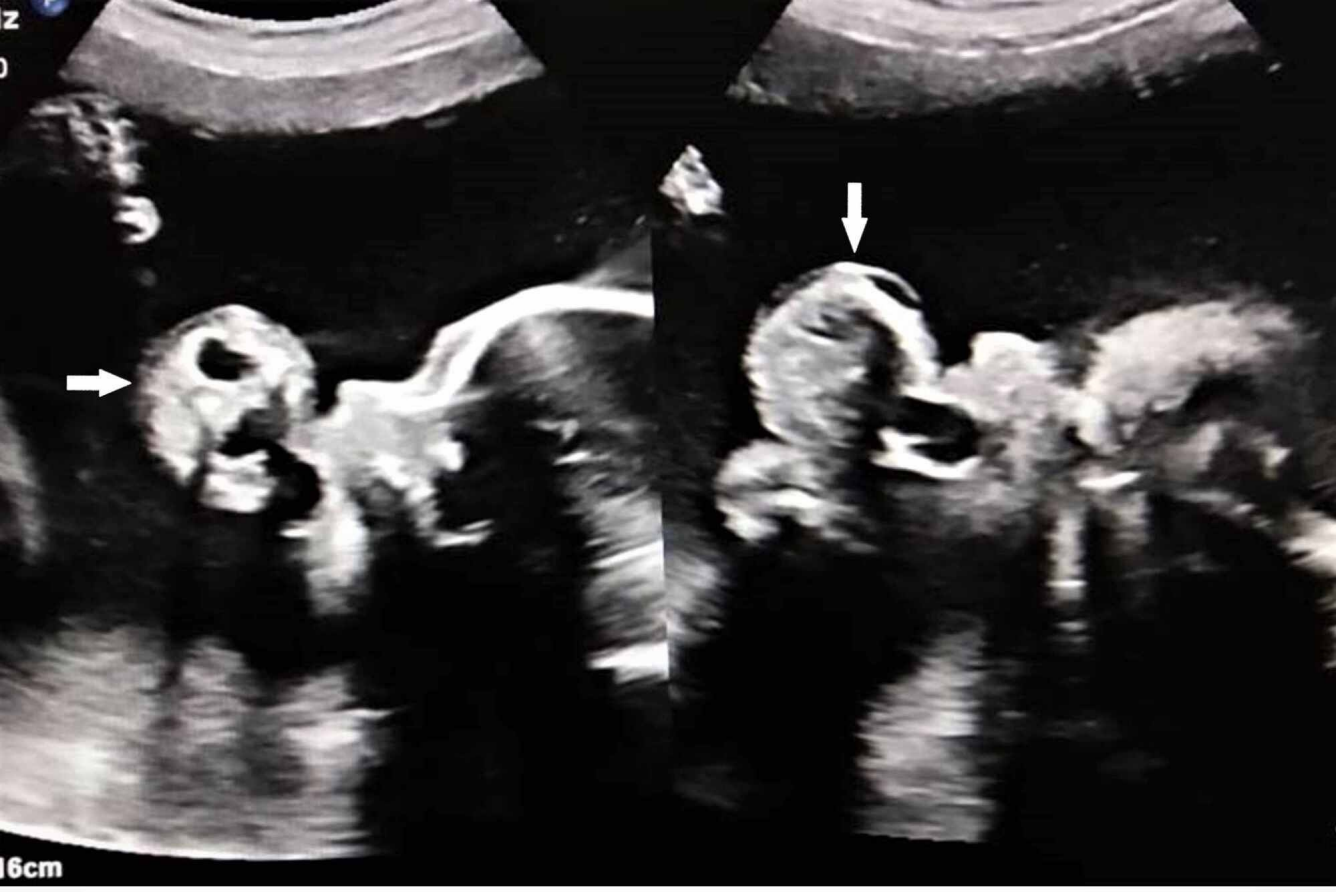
Ultrasound showing a pedunculated polypoidal mass protruding from the mouth of the fetus and freely floating in the amniotic cavity. The mass has mixed solid-cystic echotexture with areas of calcifications and minimal vascularity.
